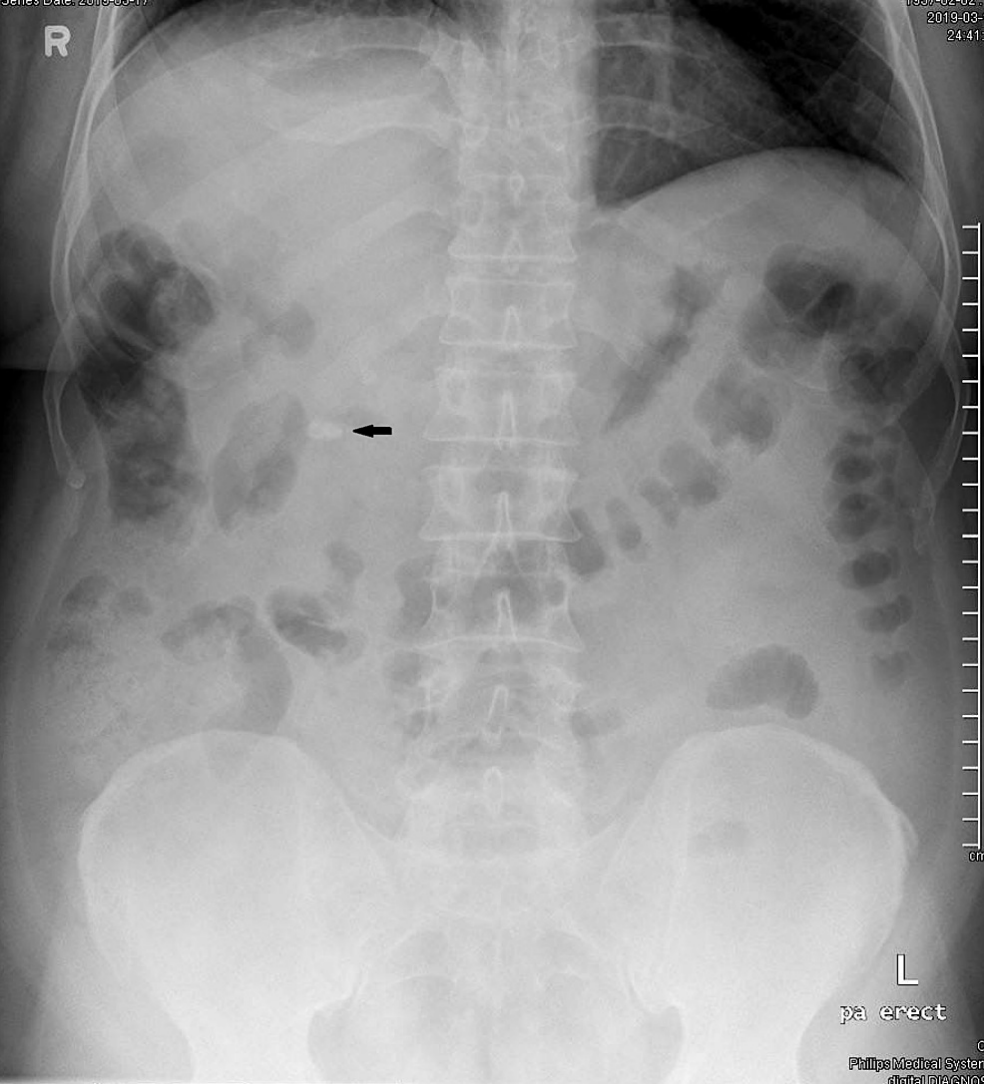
Plain abdominal x-ray showing the radio-dense shadow (black arrow) in the right upper quadrant of the abdomen. Colonic shadow is higher than usual.
